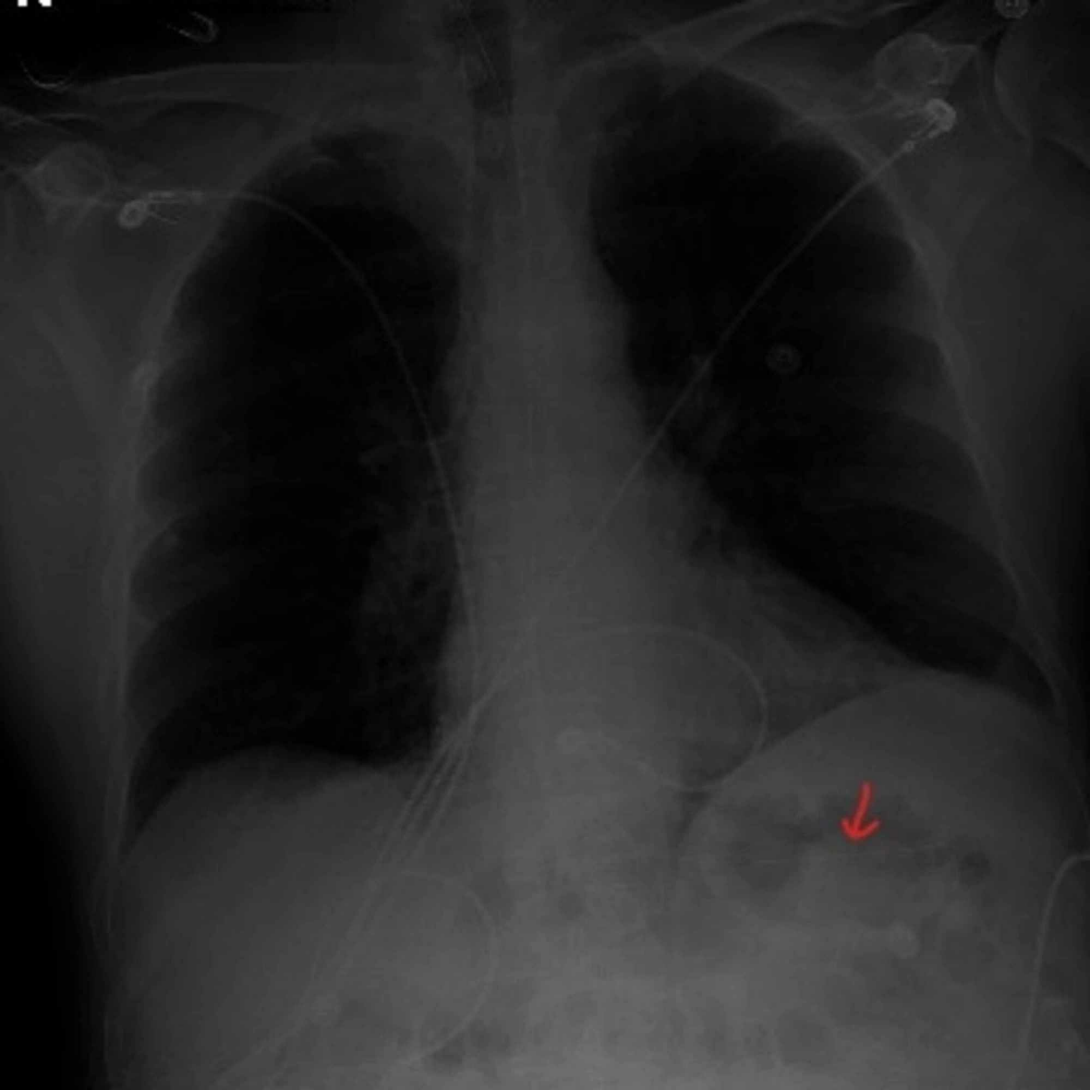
Chest X-ray confirming nasogastric tube within the stomach.
